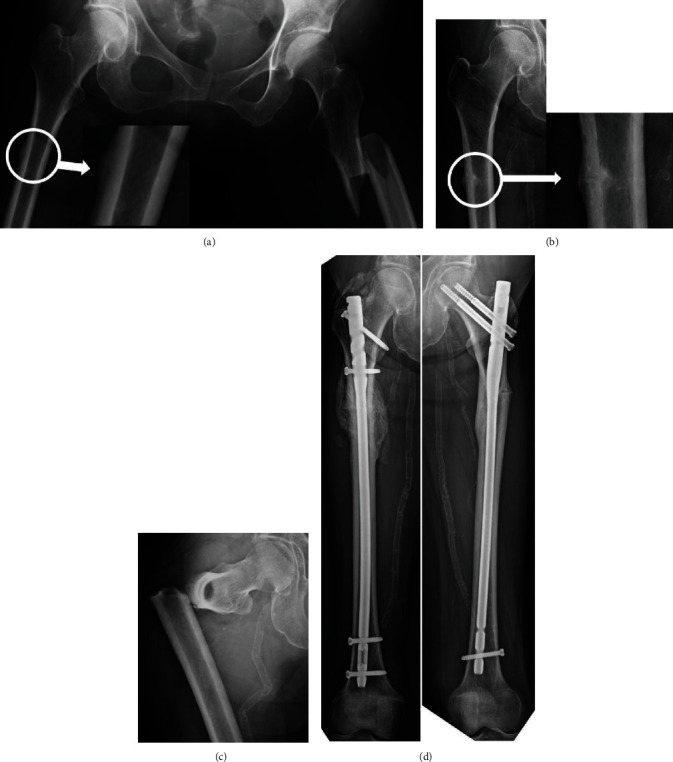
(a) The patient suffered a subtrochanteric AFF on the left side by sprain. At that time, the right femoral roentgenogram showed periosteal thickening of the lateral cortex at the subtrochanter (circle; see magnification). (b) Periosteal thickening of the lateral cortex progressed to incomplete fracture (circle; see magnification). (c) Incomplete fracture progressed to complete fracture by falling. (d) Complete bone unions were achieved on the left and right sides at 58 and 25 months after the surgery, respectively.
